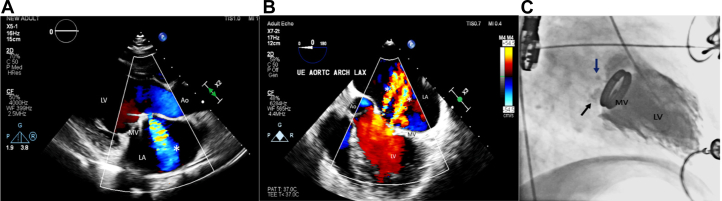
TTE, TEE, and Fluoroscopic Images (A-C): Preoperative transthoracic echocardiography (TTE), transesophageal echocardiography (TEE) images, and intraprocedural fluoroscopic images. (A) TTE parasternal long-axis view showing severe mitral regurgitation arising from aorto-mitral continuity. (B) TEE midesophageal 5-chamber view showing 2 paravalvular leak jets arising from aorto-mitral continuity (2 white asterisks). (C) Left ventricular angiogram (RAO 30° view) demonstrating 2 paravalvular leak jets (blue and black arrows). Ao = aorta; LA = left atrium; LV = left ventricle; MV = mitral valve; RAO = right anterior oblique.
